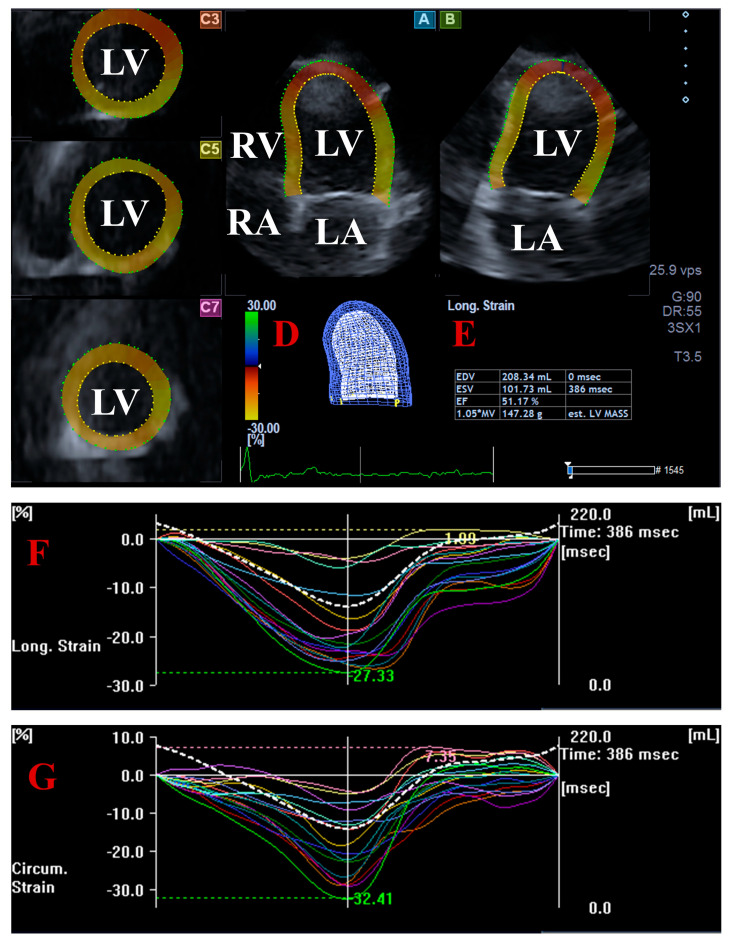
Measurement of left ventricular (LV) strains by three-dimensional (3D) speckle-tracking echocardiography: apical four-chamber (A) and two-chamber (B) long-axis views and short-axis views at apical (C3), midventricular (C5), and basal LV level (C7) are presented with a virtual 3D LV cast (D) and LV volumes and ejection fraction (E). Global (white line) and segmental (colored lines) time-longitudinal (F) and circumferential (G) time-LV strain curves are demonstrated together with a time–LV volume changes curve (dotted white line). Abbreviations: LV, left ventricle; RV, right ventricle; LA, left atrium; RA, right atrium; EF, ejection fraction; EDV, end-diastolic volume; ESV, end-systolic volume.
